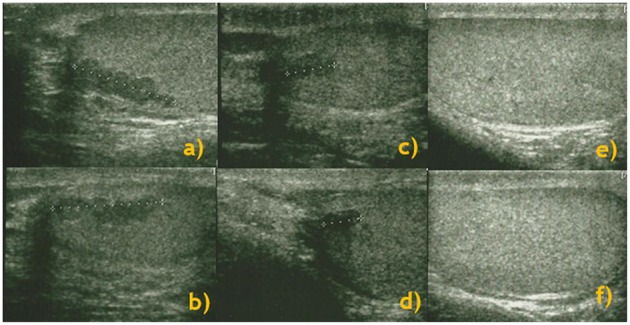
Longitudinal study: testis ultrasound performed at baseline [right (a) and left (b) testes], and 3 months [right (c) and left (d) testes] and 6 months [right (e) and left (f) testes] after cortisone therapy and a consequent improvement in ACTH levels.
